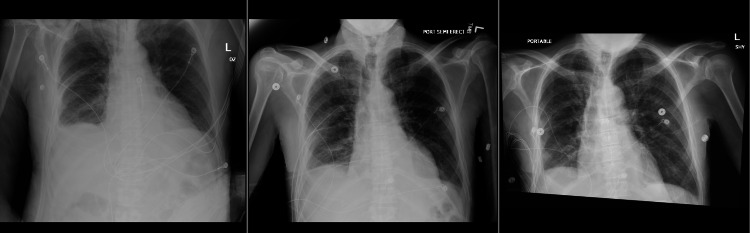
Serial chest X-ray post-chest tube removal showing improvement in pleural effusion (left to right)
